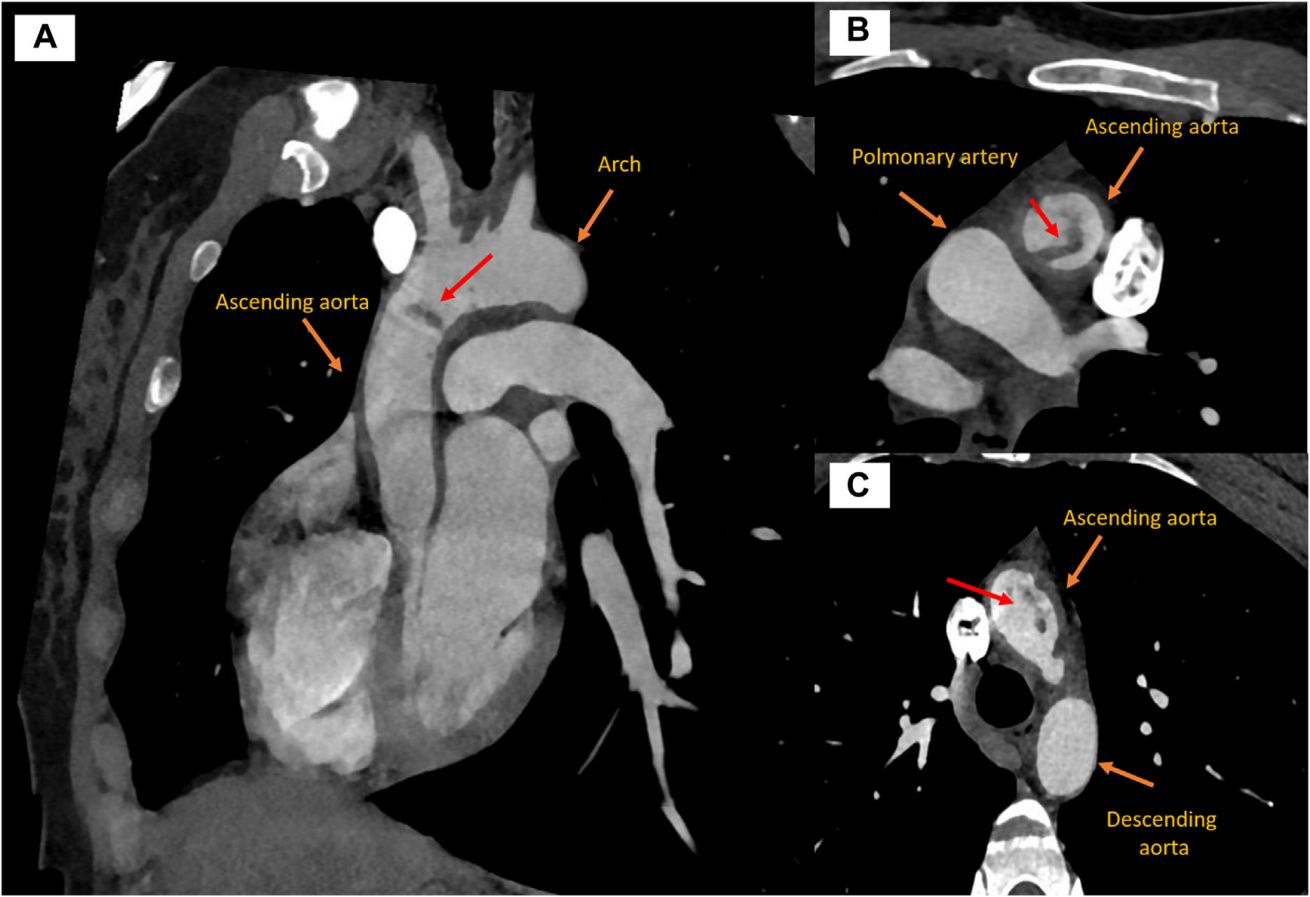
Computed Tomography Images (A to C) Images show the arch, ascending aorta, and descending aorta indicated by orange arrows, while the lesion in the ascending aorta is indicated by a red arrow.
